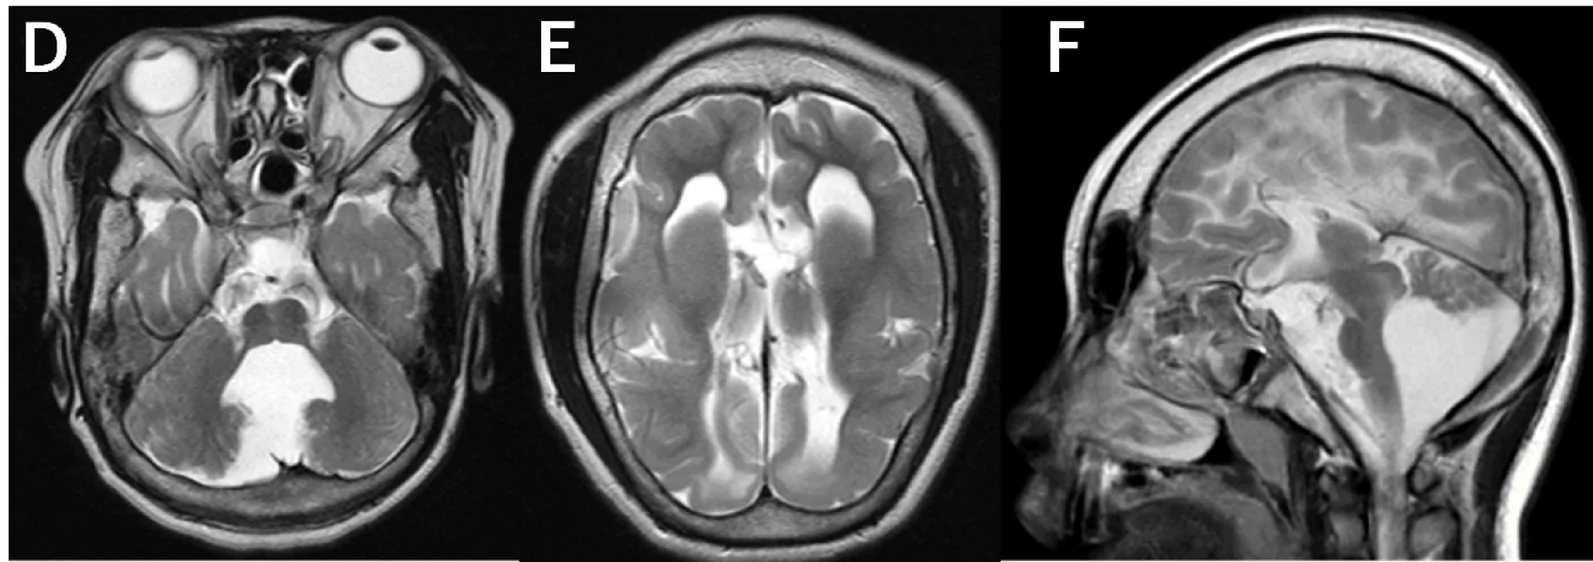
Brain MRI findings of Case 2 with Dandy–Walker malformation. D, E: T2-weighted axial images, and F: T2-weighted sagittal images at age 13. These images show complete cerebellar vermis defect (D arrow), basal ganglia malformation (E arrow), corpus callosum dysplasia (F arrow), and a giant cyst contiguous to the fourth ventricle (F white arrowhead)
